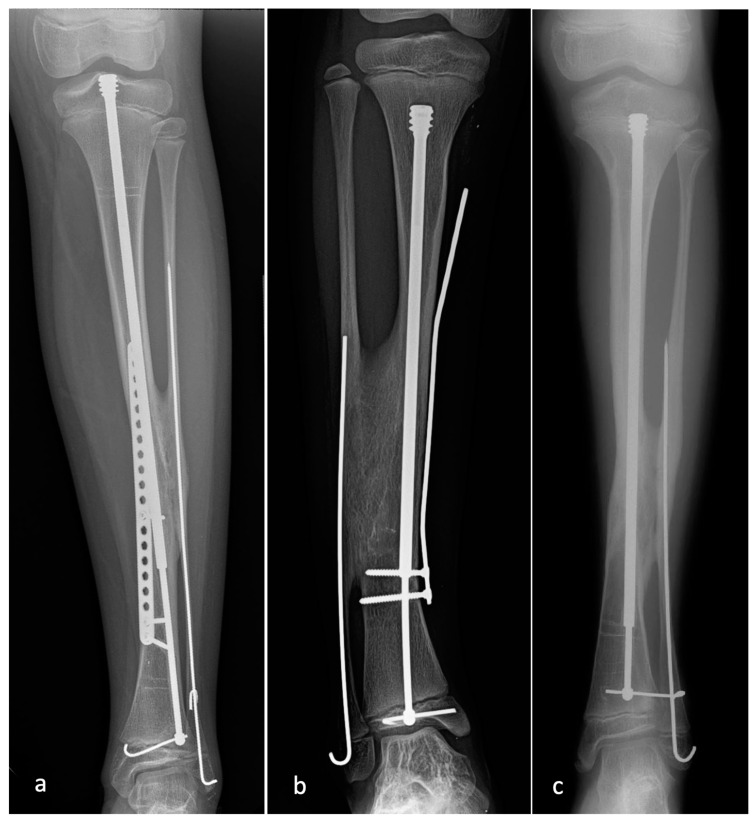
Radiographs demonstrating the various forms of FD rod migration: (a) the rod has pulled out of the distal epiphysis; (b) the rod has pulled out of the proximal epiphysis; (c) the rod has pulled out of both proximal and distal epiphyses. Note the reconstitution of the physis despite the breach by the FD rod.
